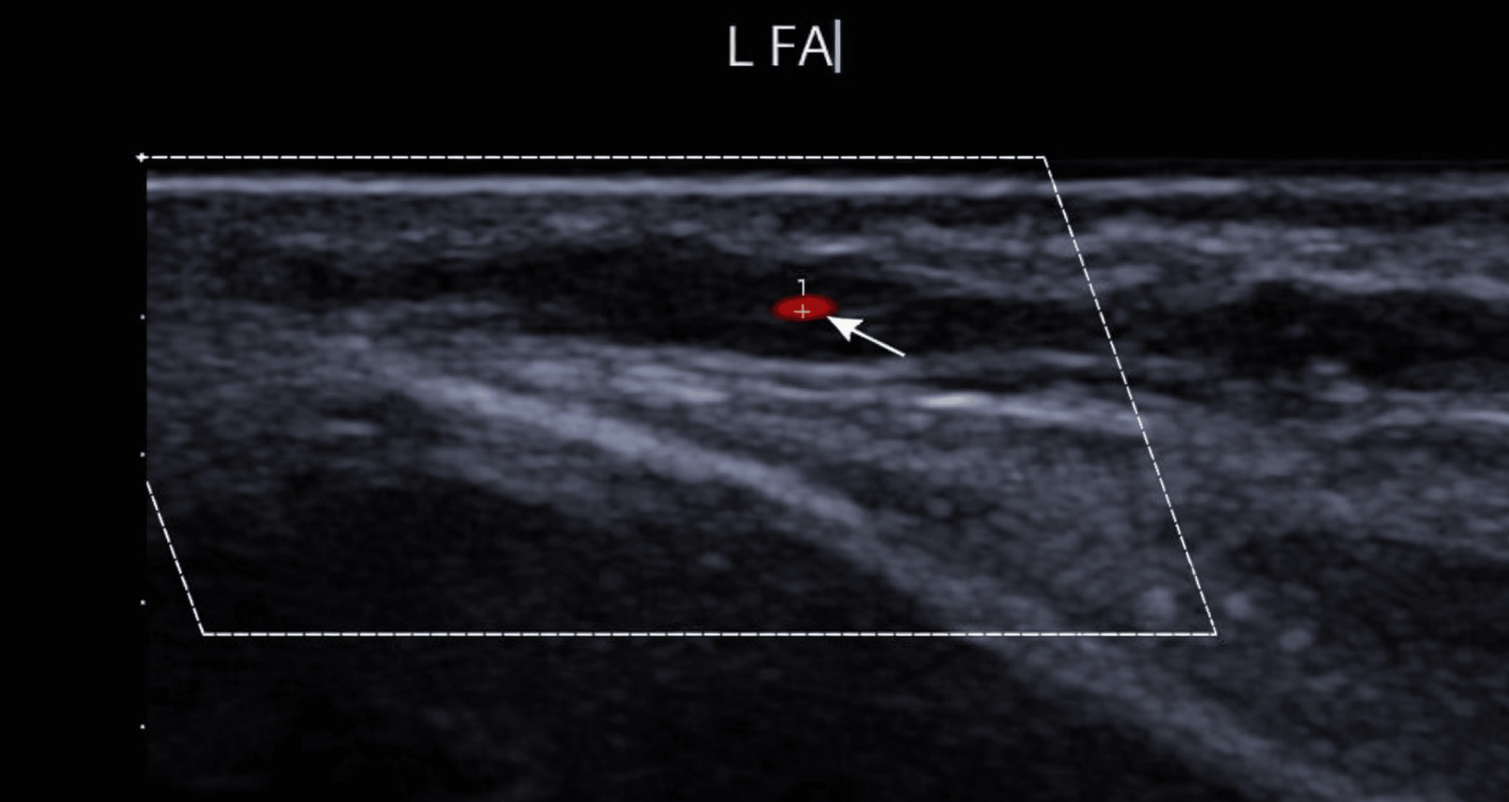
Temporal artery ultrasound demonstrating a halo sign consistent with giant cell arteritis
